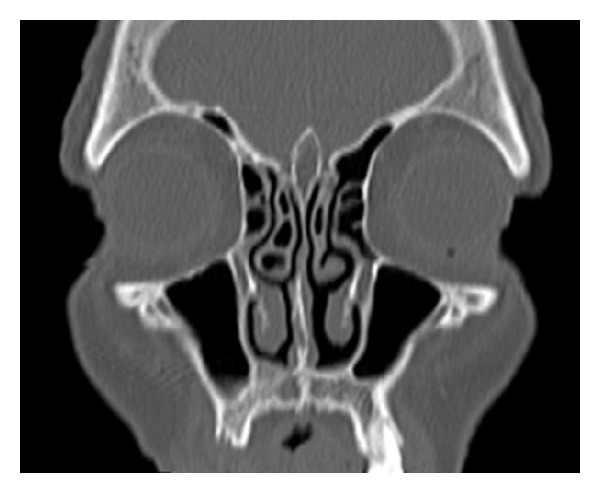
Coronal CT scan showing two thin bony septums inside the CB cavity and hypertrophy of inferior turbinate in the right side, besides lamellar type CB in the left side.
